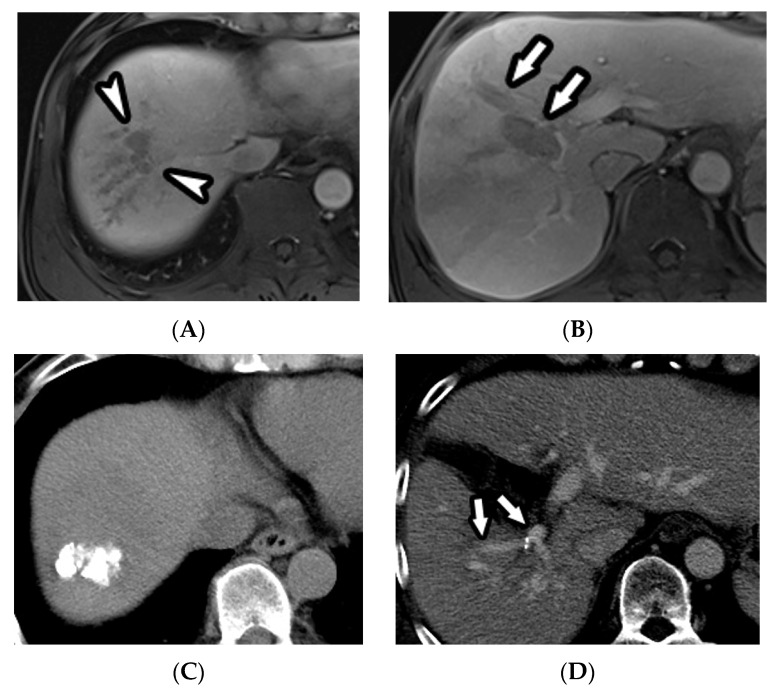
(A) A 51-year-old male patient with HCC and lobar PVTT. (A,B) Contrast-enhanced axial MRI images in the venous phase show a small HCC (3.4 cm maximum diameter, arrowheads) with right lobar PVTT (arrows). (C,D) Six months after TACE plus RT and additional TACE, non-contrast enhanced (C) and contrast-enhanced axial CT (D, in the venous phase) images show a compact lipiodol uptake in the tumor (C) and disappearance of the right lobar PVTT (D, arrows), with a decrease in volume of the right hemiliver.
